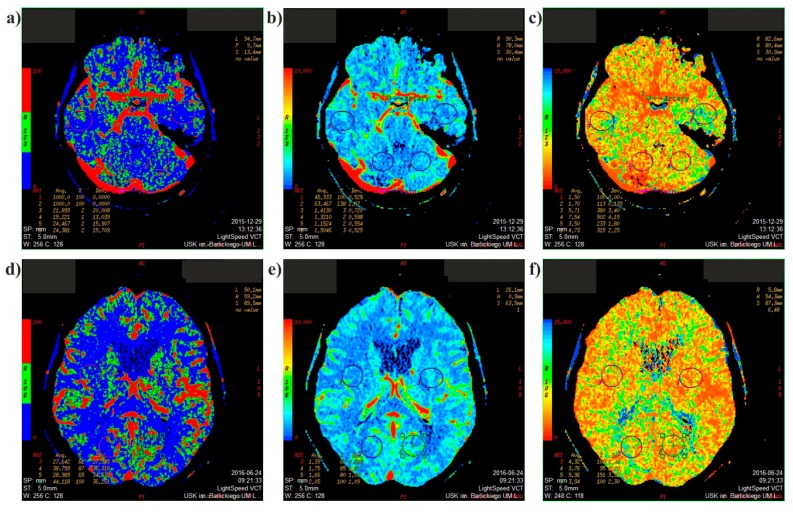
CT-perfusion after surgical intervention, axial scans: (a) blood flow—before; (b) blood volume—before; (c) mean transient time—before; (d) blood flow—after; (e) blood volume—after; (f) mean transient time—after.
